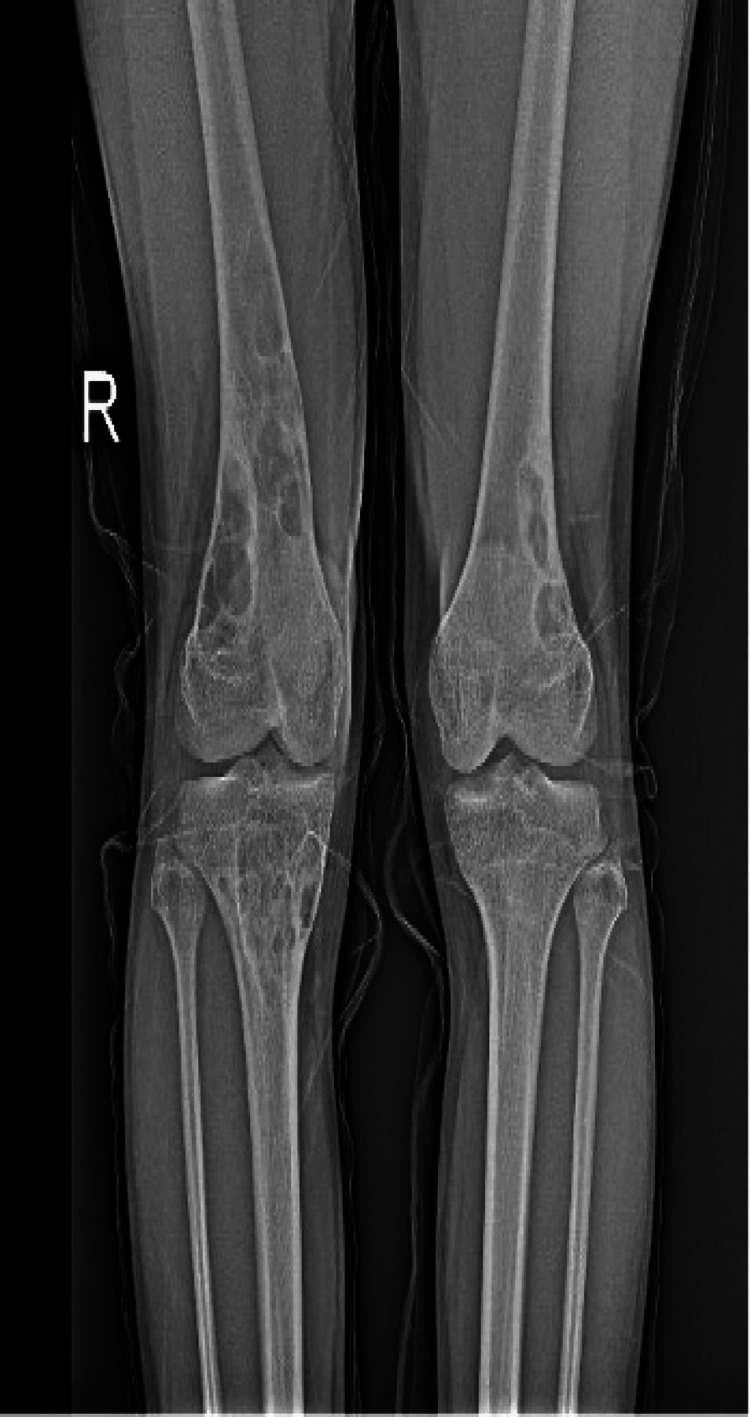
Radiographs showed multiple NOFs NOFs: non-ossifying fibromas
